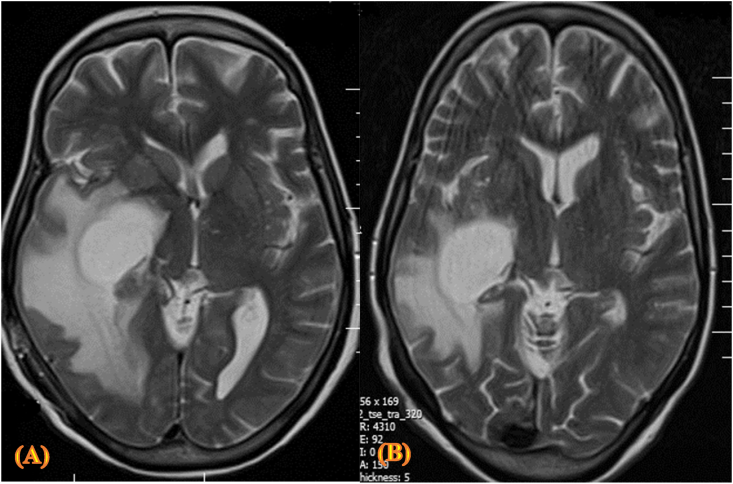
A. One-month postoperative MRI image is shown. Axial view of T2W: Edema persists, but the midline shift has decreased since the start of amphotericin B; B. Four-month postoperative MRI image. T2W Axial view: After 3 months of amphotericin B and voriconazole, edema and midline shift were reduced. (MRI, magnetic resonance imaging).
